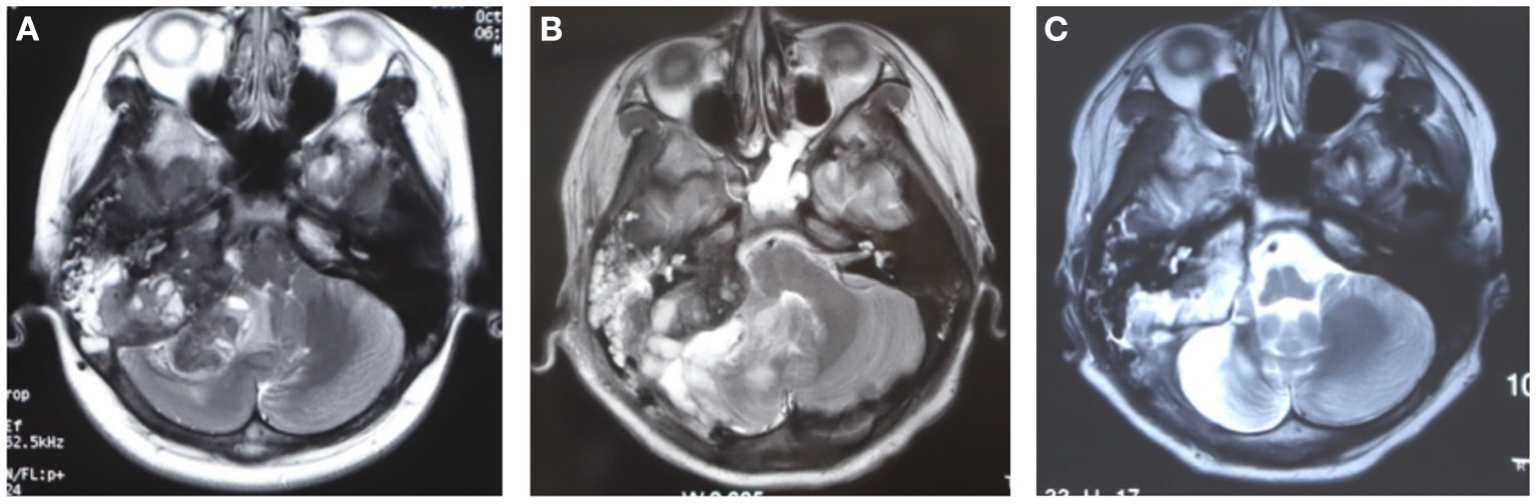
The brain MRI images before and after primary surgery. (A) The preoperative image showed a tumor occupied in the posterior fossa, medial to the right mastoid bone. (B) The postoperative 9 days MRI after surgery showed edema in the surgical region, some residual tumor existed for being unable to resect the tumor completely. (C) The MRI image showed that the tumor remained stable 6 months after radiotherapy.
